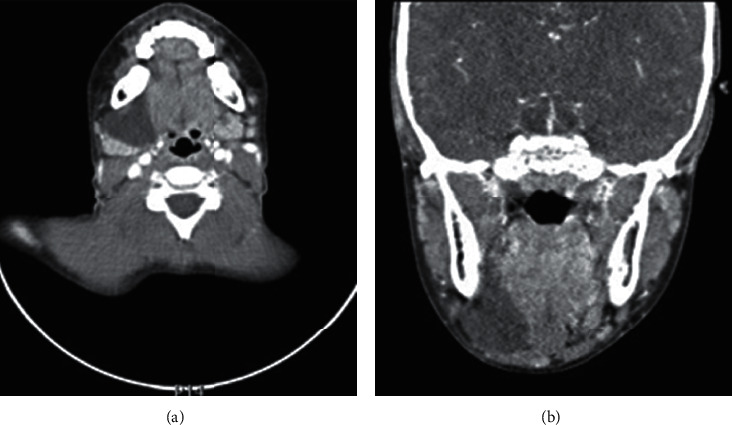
(a, b) Injected cervicofacial CT-scan demonstrating the presence of a well-delineated liquid lesion of 25 × 35 × 29 mm.
